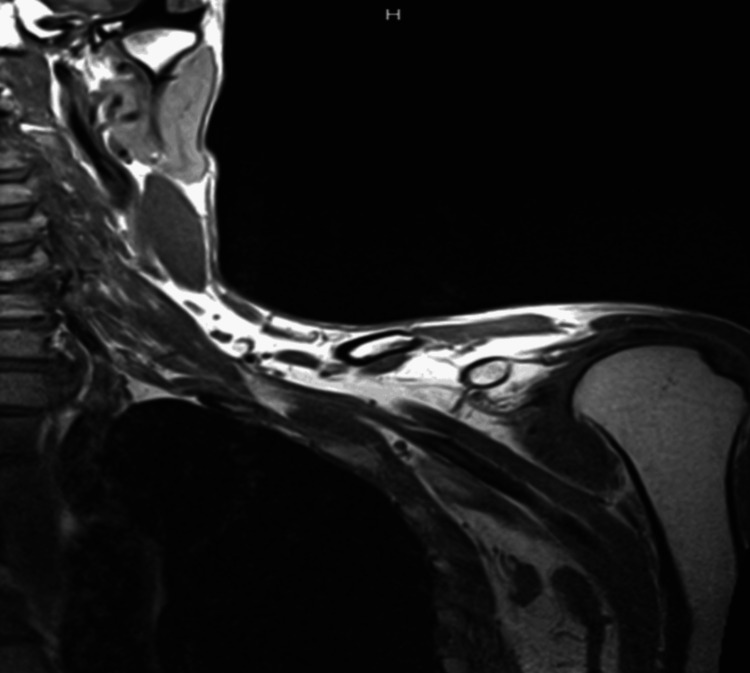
MRI of brachial plexus showing no T2 signal abnormality or regional abnormal enhancement. No invading mass or focal abnormality was observed in the lung apex or regional soft tissues. MRI: Magnetic resonance imaging
